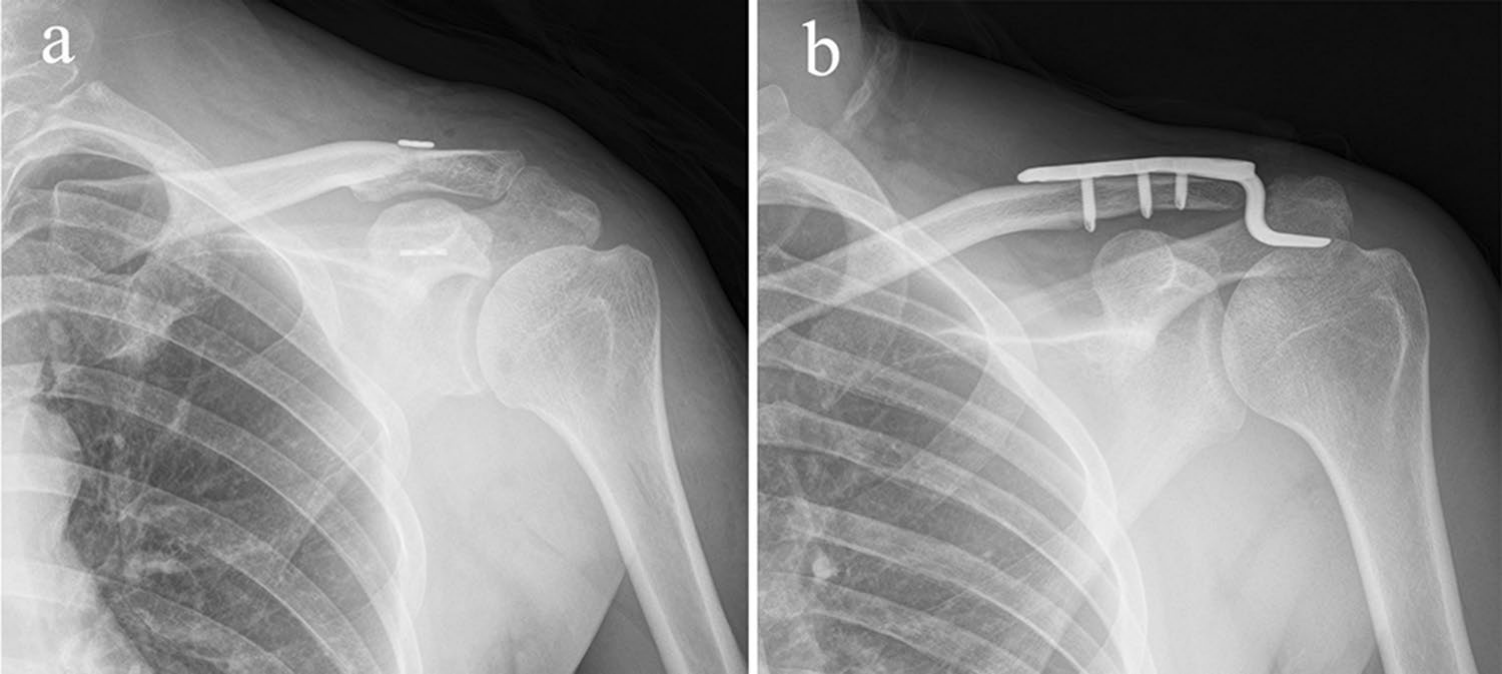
Radiographs immediately after the surgical procedures. (a) MITR fixation. (b) HP fixation.
